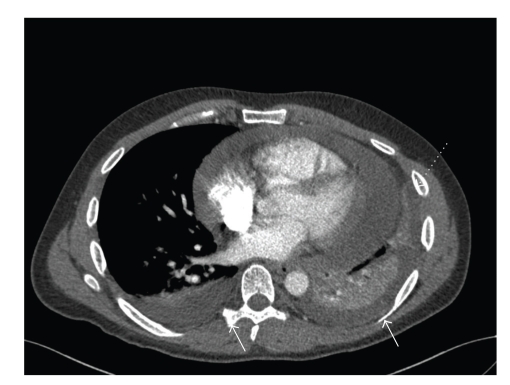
CT scan showing pericardial (dashed arrow) and bilateral pleural effusion (solid arrows).
